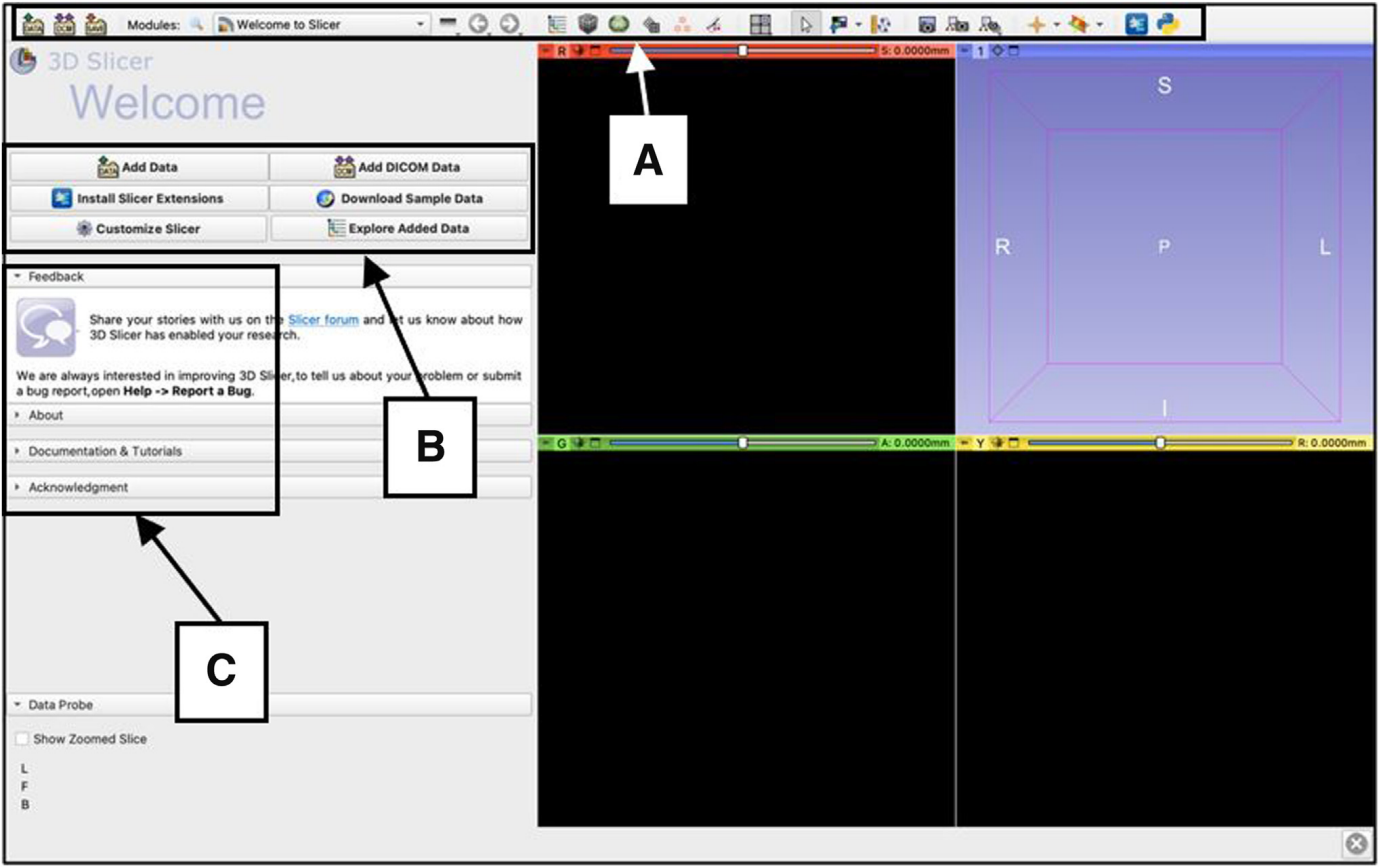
Standard display of the home page of the 3D Slicer application on a computer with macOS software. This is the starting point that is seen before using the application for the manual segmentation of magnetic resonance imaging to create a 3-dimensional reconstruction. The toolbar on the screen can also be used to load and save data, change modules, change views, screenshot image views, along with a variety of other functions (A). New and sample data can be loaded, and extensions can be installed from this page (B). Documentation and tutorials on how to use the program, along with other informational sections, are also available (C).
